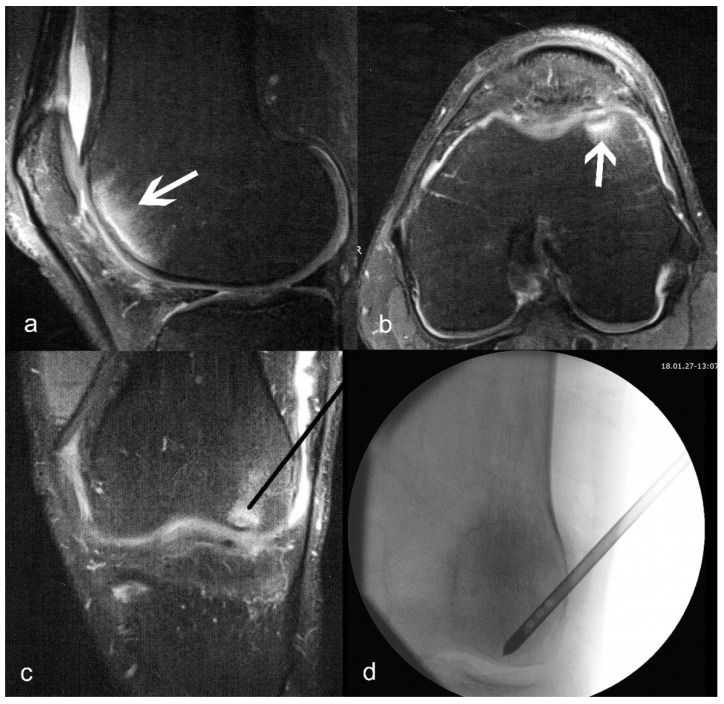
(a) Sagittal and (b) axial T2-weighted images of bone marrow lesion (shown with arrows) of the medial condyle; (c) planned position of the delivery canula placement (black line); (d) actual fluoroscopy indicating correct placement of the delivery canula.
